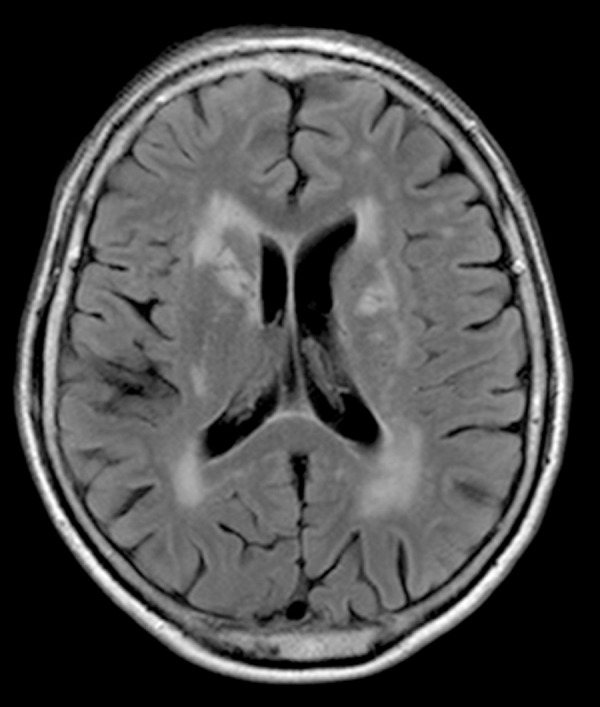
Flair MRI revealing moderate-severe lesions in white matter.
